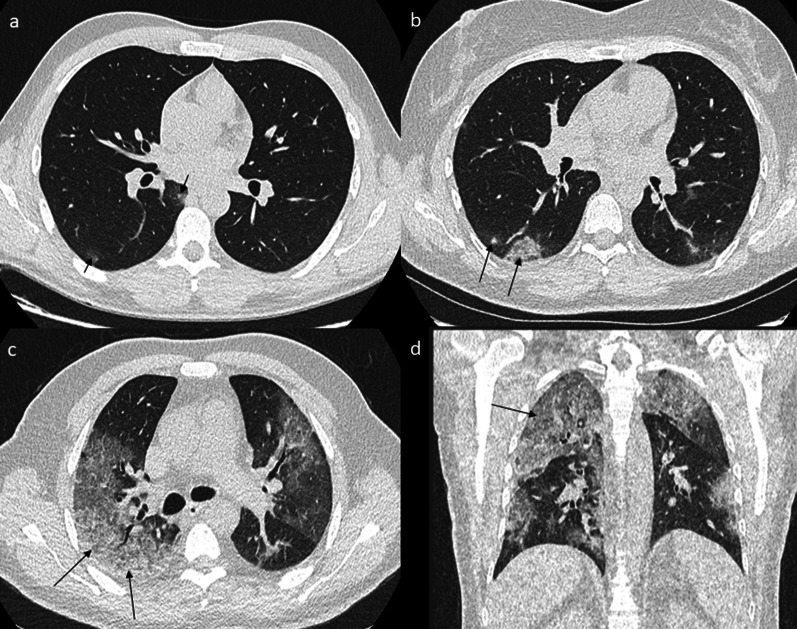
CT-SS, instances of different involvements. Note: a A 27-year-old male COVID-19 patient with no medical history presenting cough, anosmia and fever for 4 days. LDCT shows two GGOs in the right 6th segment (arrow) corresponding to a minimal impairment with the presence of < 10 secondary lobules. The global CT-SS was 1. b A 44-year-old female COVID-19 patient with no medical history presenting cough, rhinorrhea and myalgia for 5 days. LDCT shows GGO in the right 6th segment (arrow) corresponding to an intermediate impairment with the presence of < 50% involvement of the 6th segment. The global CT-SS was 4. c, d A 65-year-old male COVID-19 patient with diabetes and hypertension presenting cough, rhinorrhea, anosmia, myalgia and dyspnea for 7 days. LDCT shows GGO and partial consolidation in the right 2nd segment (arrows), corresponding to severe impairment with more than 50% involvement of the segment. The global CT-SS was 30
